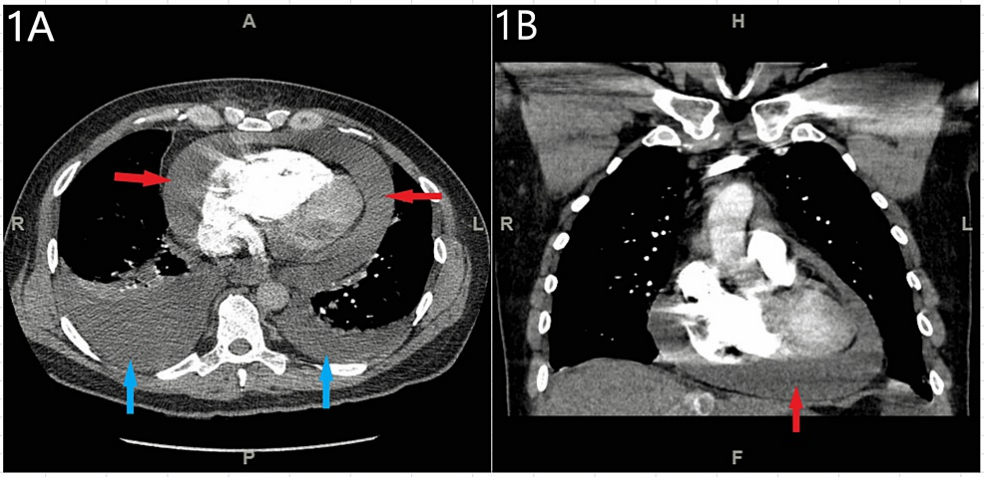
CT angiography of the chest showed large pericardial effusion (red arrows), large right and moderate left pleural effusions (blue arrows). Figure 1A - axial view, Figure 1B - coronal view
